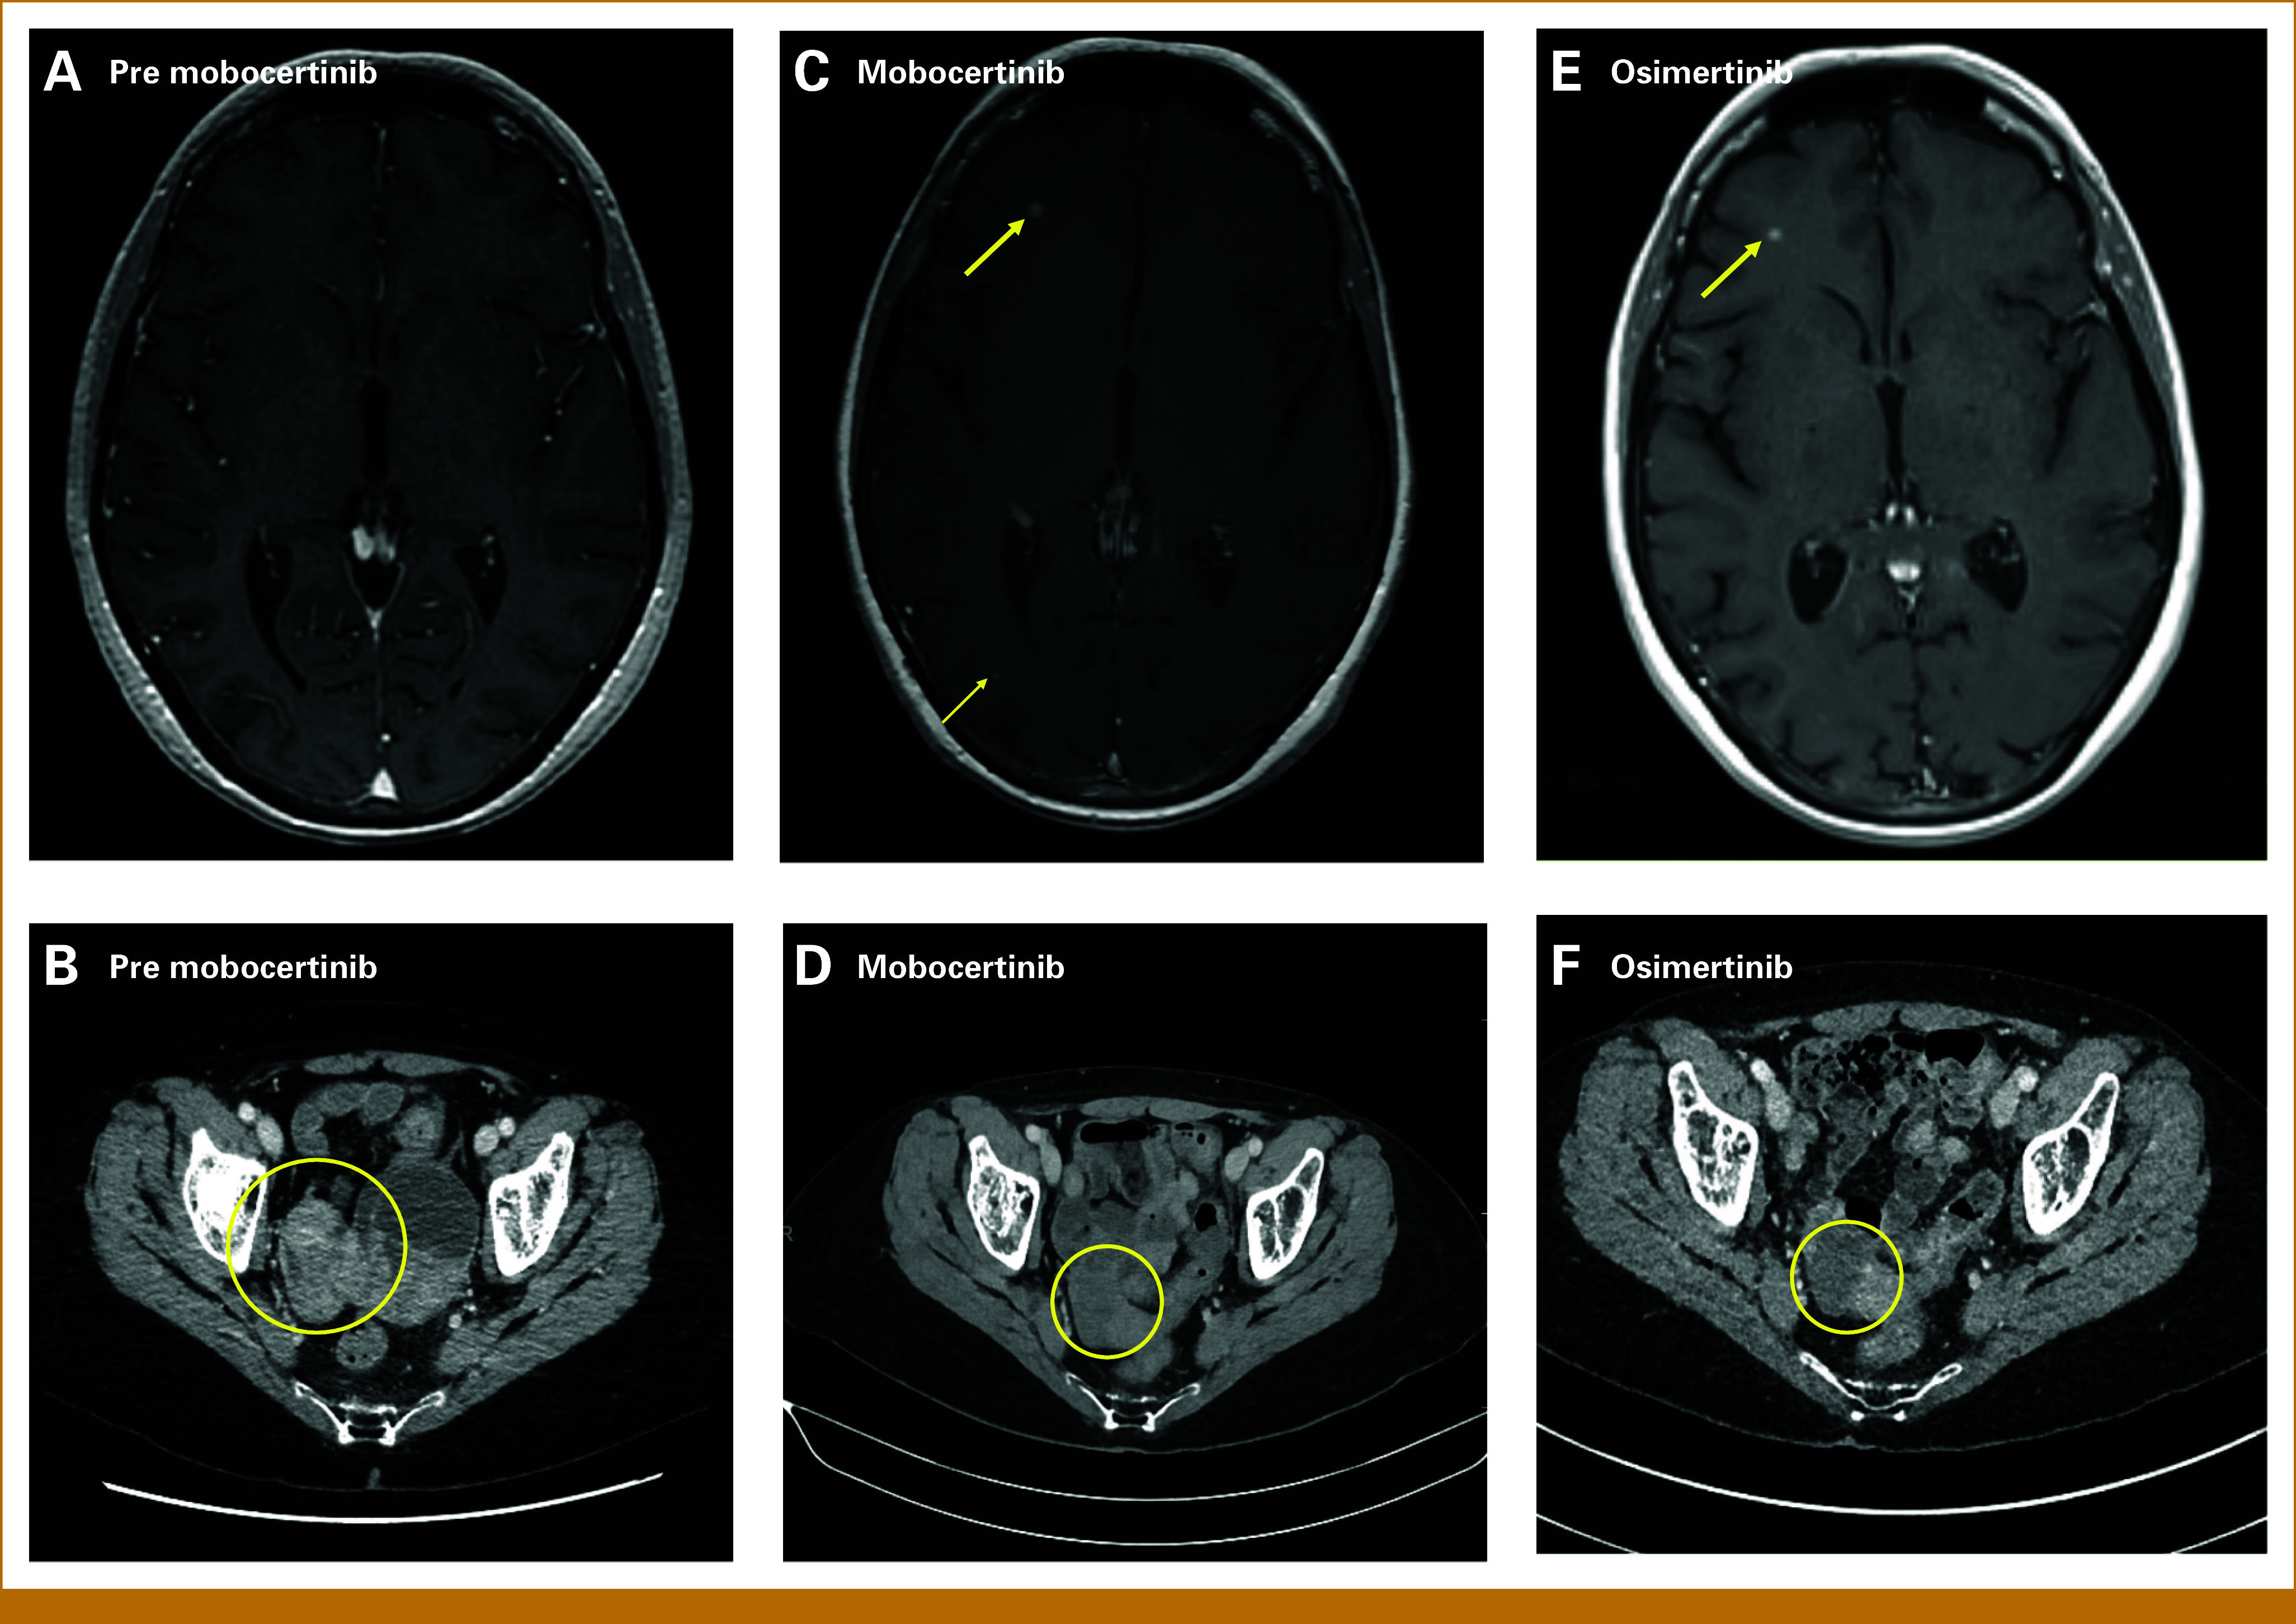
Images demonstrating treatment responses to mobocertinib and osimertinib. (A) Brain MRI 2 months before starting mobocertinib shows no intracranial disease. (B) Chest, abdomen, and pelvis CT before mobocertinib shows an enlarged right ovarian metastasis. (C) Brain MRI after 6 months on mobocertinib shows intracranial progression. Dozens of small, enhancing intraparenchymal foci were detected, predominantly within the supratentorial compartment. Two lesions (yellow arrows) are observed in the image frame. The largest lesion, detected at the gray-white junction of the anterior-inferior right frontal lobe, reached a maximum dimension of 6 mm (thick yellow arrow). (D) PET/CT after 8 weeks on mobocertinib demonstrates a partial response (–62% reduction in largest diameter) within right ovarian metastasis. (E) Brain MRI after 8 weeks on osimertinib shows intracranial response and a residual enhancing focus within the right frontal lobe (thick yellow arrow). (F) Initial chest, abdomen, and pelvis CT on osimertinib shows disease control of the right ovarian metastasis. MRI, magnetic resonance imaging; PET/CT, positron emission tomography/computed tomography.
